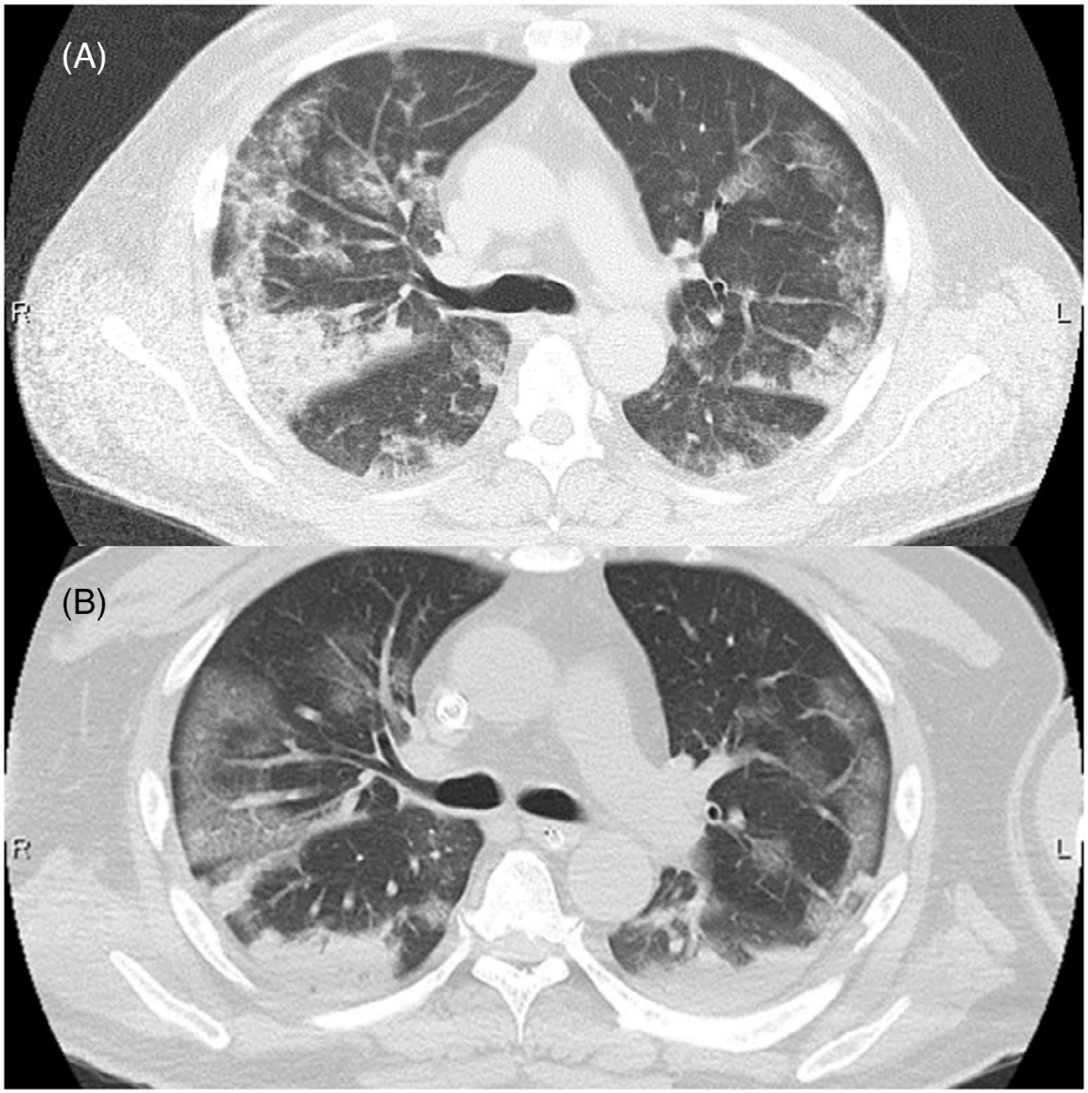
(A) An axial thoracic CT image obtained soon after recovery showing bilateral patchy ground‐glass opacities and accented non‐segmental shadows in the subpleural region. (B) An axial thoracic CT image obtained 4 months previously, immediately after intubation for ECMO therapy, showing typical imaging findings of SARS‐CoV‐2 pneumonitis with ARDS
